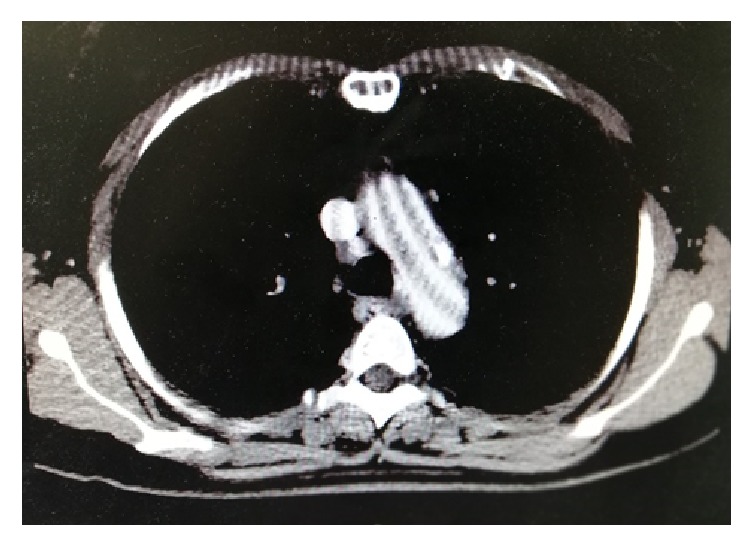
CT angiography revealed dislodgement of aortic thrombus and occlusion of the left common femoral artery (Absence of contrast agent in the arterial phase).
